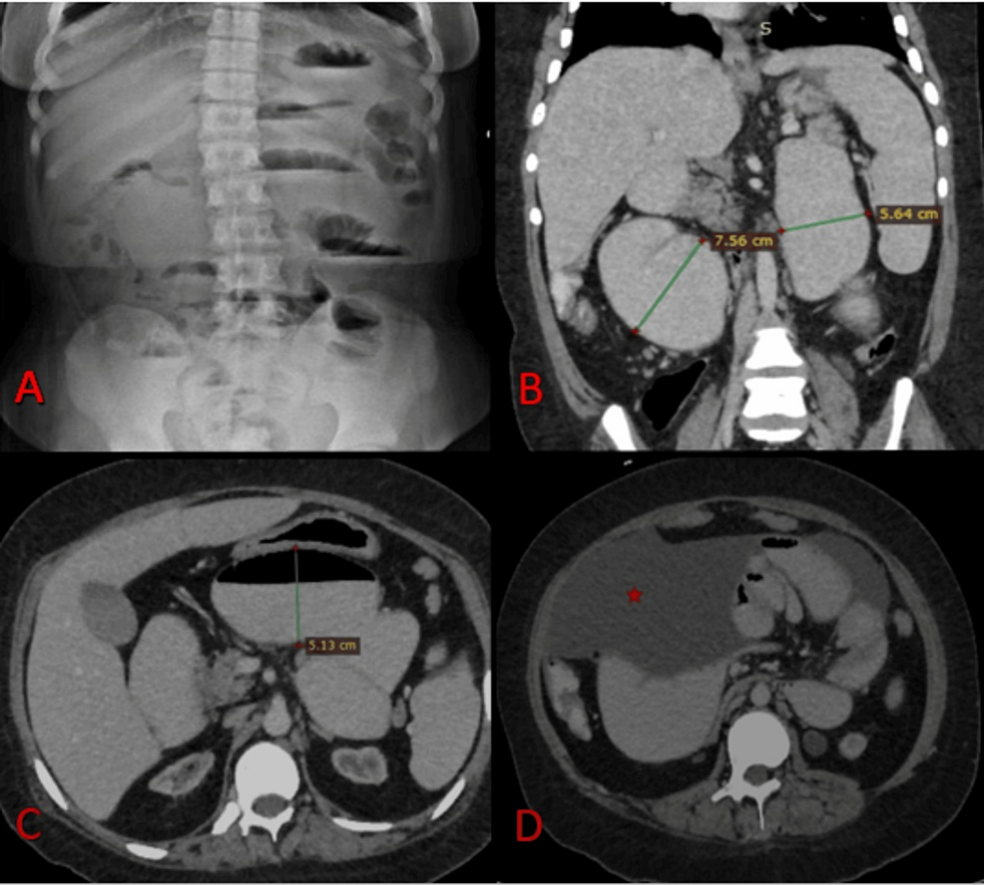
Imaging of the patient at the time of admission A: A standing abdomen x-ray reveals multiple air-fluid levels, indicating an intestinal obstruction; B and C: CT of the abdomen, showing dilated small bowel loops in the coronal and axial sections, respectively; D: Axial view of the abdomen CT reveals free fluid in the abdomen (red star)
